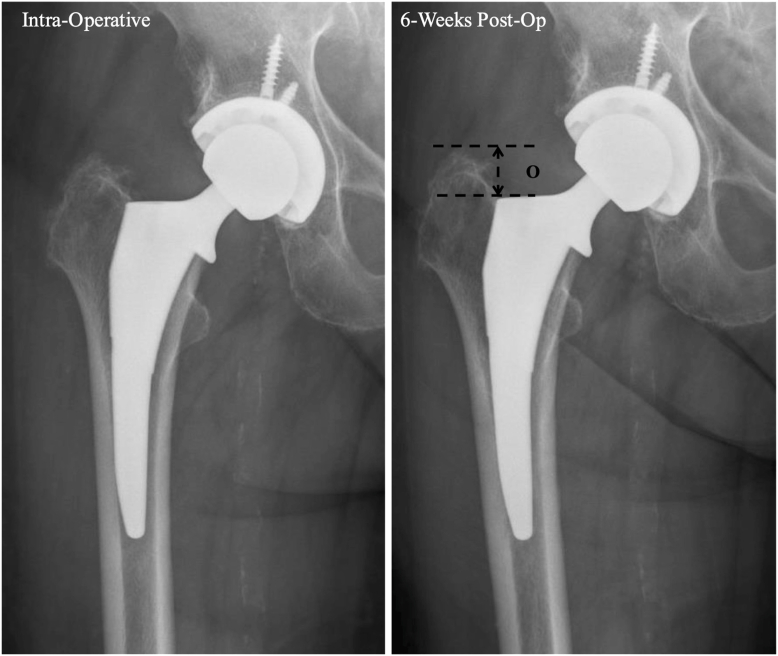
Radiographic method for measuring stem subsidence. Stem subsidence was defined as the vertical shift of the stem > 3 mm inside the femur within the first 6 weeks postoperatively. Stem subsidence was measured by assessing the distance between 2 fixed points: the tip of the greater trochanter and the flat horizontal shoulder of the prosthesis.
